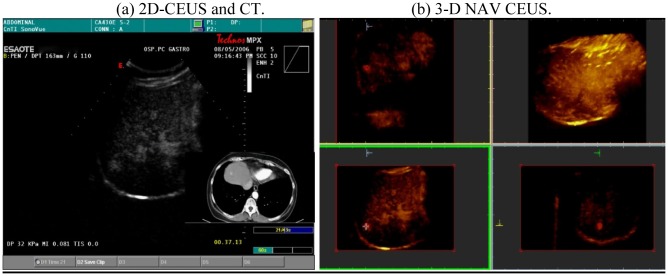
(a). Bidimensional acquisition plane of a female patient with elevated values of AFP and conventional US negative for new nodules. Only spiral CT was able to detect a small HCC in VIII segment. 3-D NAV CEUS detected the nodule; (b). The 3-D reconstruction easily showed the single nodule on the three different planes (red cross) and on the volumetric reconstruction. After the PEI treatment, AFP became normal and no new nodules were detected during the six months follow up.
